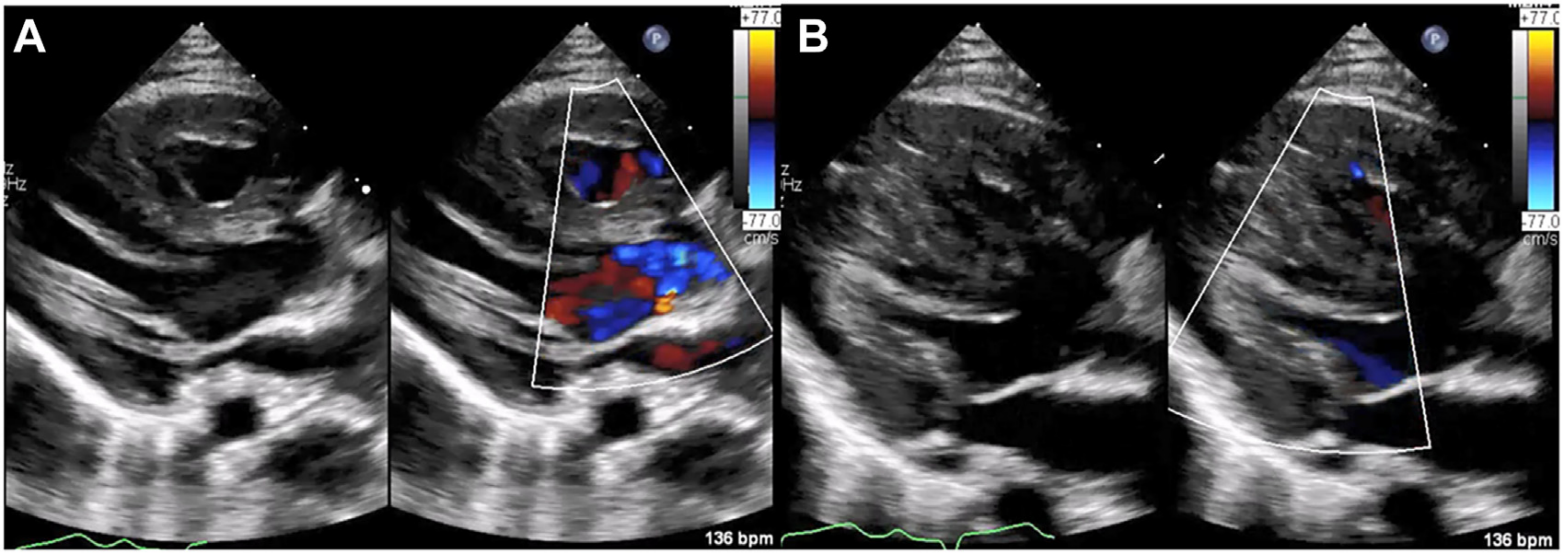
(A, B) Preoperative transthoracic echocardiography showing a hypertrophic biventricle and ventricular septum directing to the mitral valve, meeting the criteria for asymmetric ventricular septal hypertrophy.
